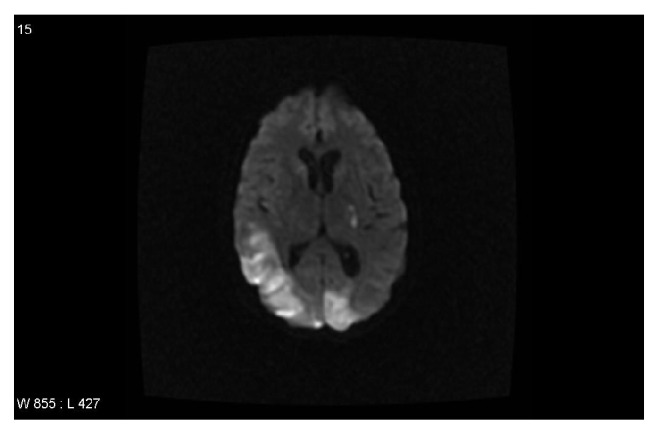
MRI brain on presentation.
